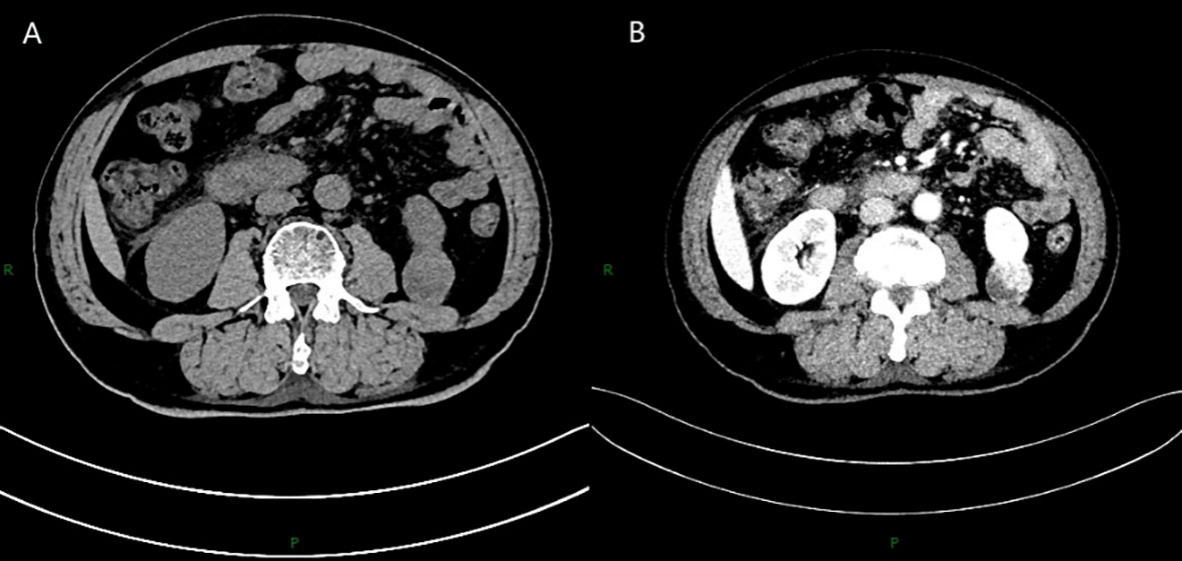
CT plain scan showed a 3.7*3.0 cm round soft tissue density focus at the lower pole of the left kidney (A), and the enhancement scan showed the lesion was inhomogeneous enhancement (B).
